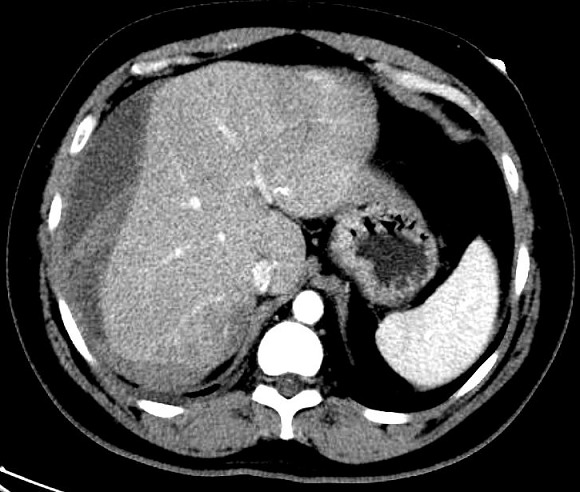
Abdominal computed tomography showing 16 x 10 x 3 cm large hepatic subcapsular hematoma and no focal lesion
